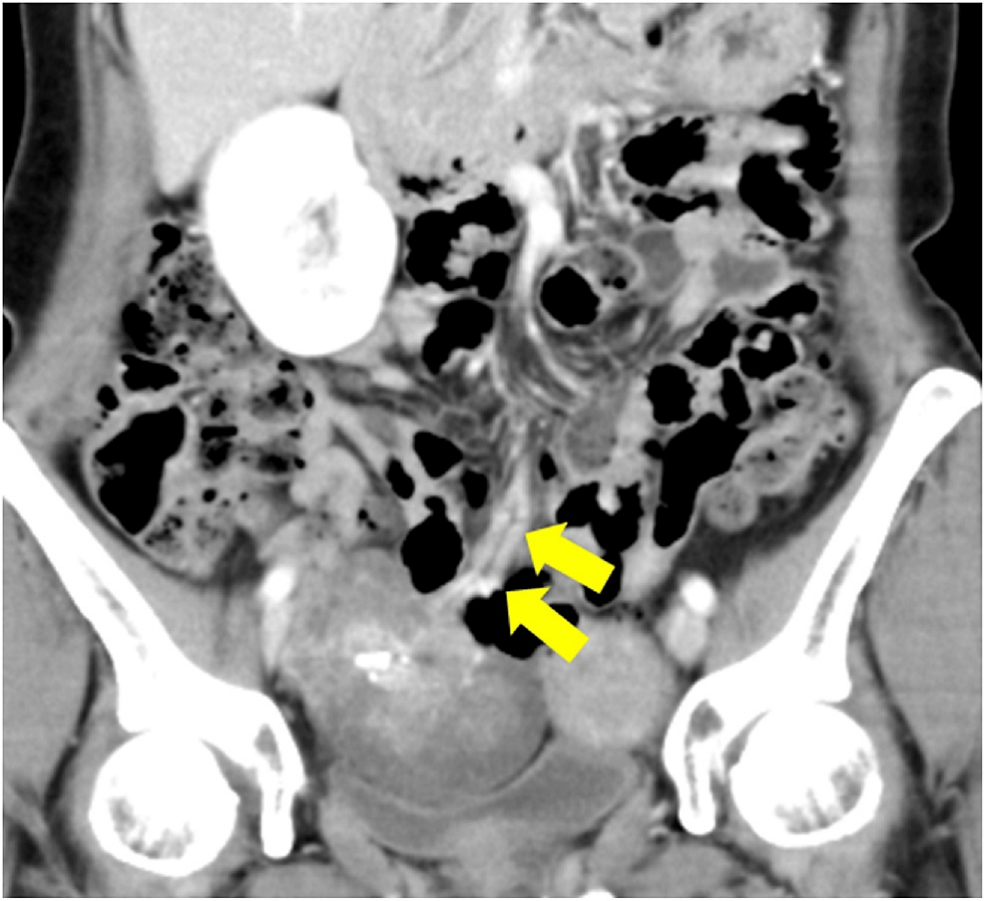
Preoperative abdominopelvic contrast CT image (coronal view). A peripheral branch of the SMV mesenteric vein flowed into the mass. SMV: Superior mesenteric vein
